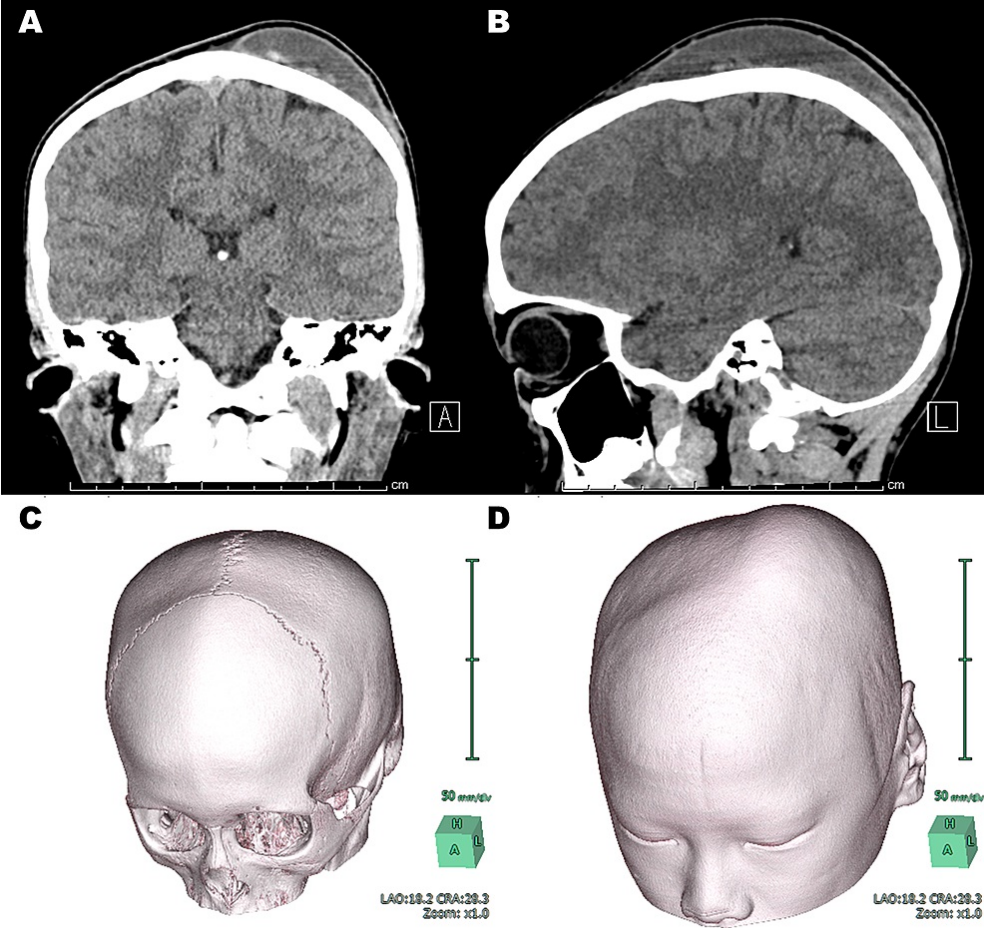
MPR images of CT scan and volume rendering. A coronal MPR image shows subcutaneous hematoma (A) as does a sagittal MPR image (B). The hematoma does not cross the suture lines (C), indicating that this subcutaneous hematoma (A, B, D) is C-SPOH. MPR, multiplanar reconstruction; C-SPOH, calvarium subperiosteal hematoma
